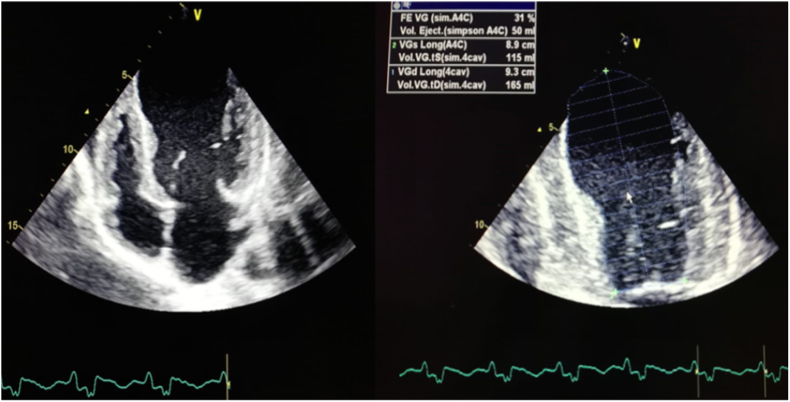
Echocardiography imaging displaying severe systolic dysfunction (EF 31%).
